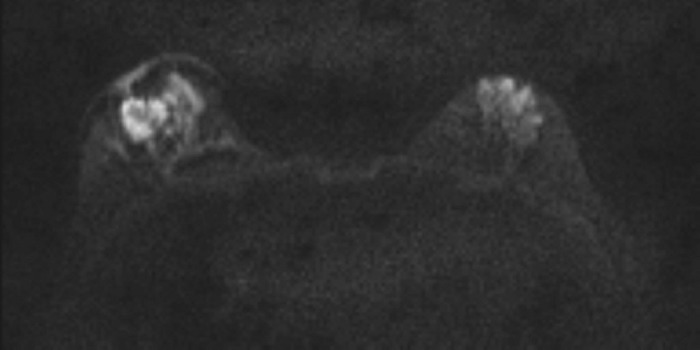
Representative case of positive DWI rim sign. Diffusion weighted image (b = 750 s/mm^2^) shows a complete high signal rim surrounding the right breast mass.
